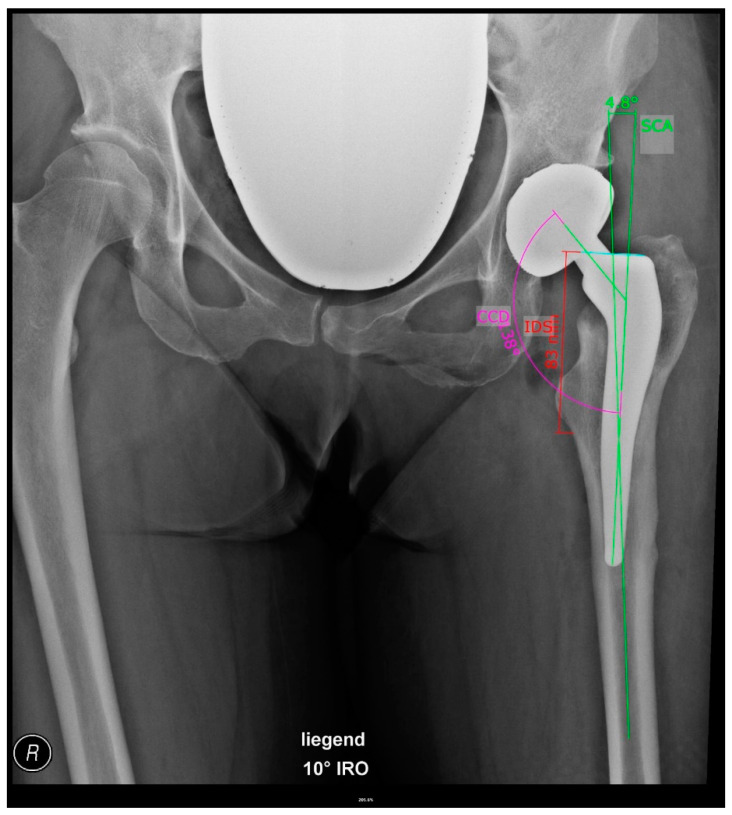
Postoperative measurement on AP pelvis X-ray in 10° internal rotation (IRO). Implantation depth of the stem (IDS), projected neck-to-shaft angle (CCD), and stem coronal angle (SCA).
